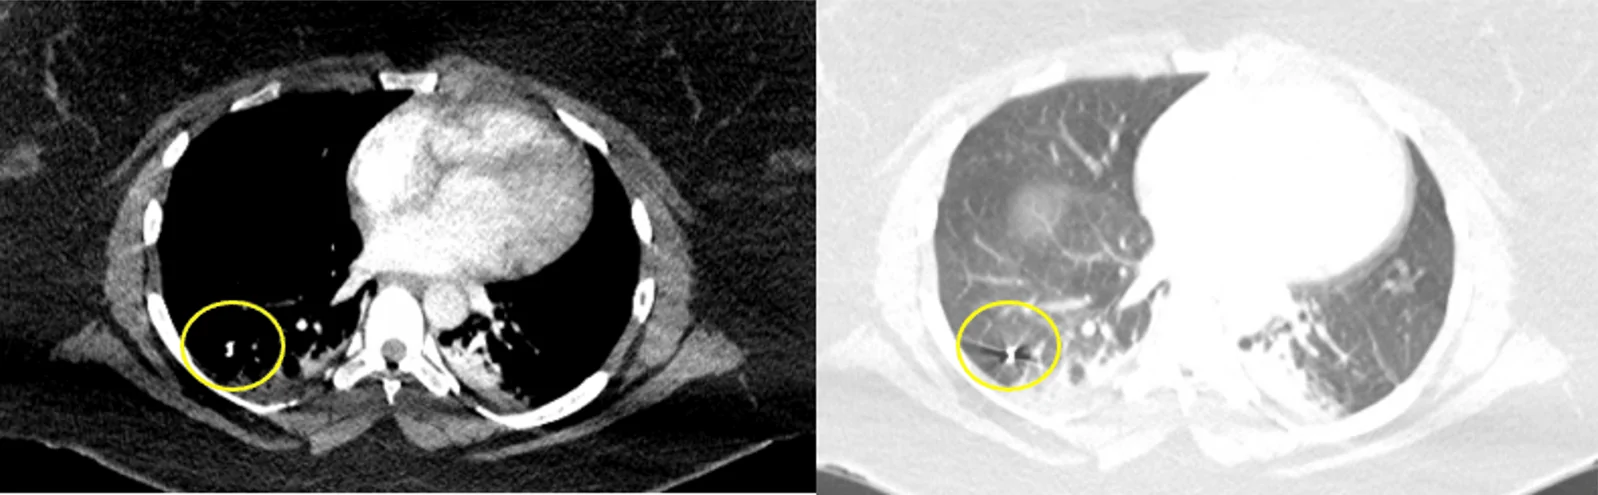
CT images in soft tissue and lung windows demonstrating a pellet in the right lung periphery. Left image: soft tissue window. Right image: lung window.
